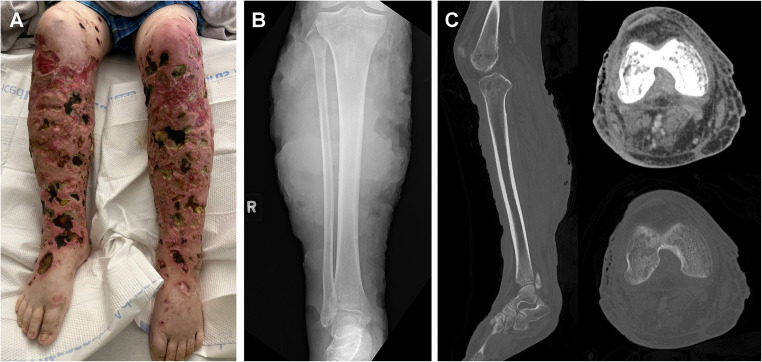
33-year-old man with (A) extensive soft-tissue wounds involving both lower extremities. (B) Radiographs of the right tibia and fibula demonstrate soft-tissue defects and subcutaneous air. (C) Contrast-enhanced CT of the right lower leg shows no gas or ulceration adjacent to the bone, no exposed bone, and no osseous erosion to indicate acute osteomyelitis
